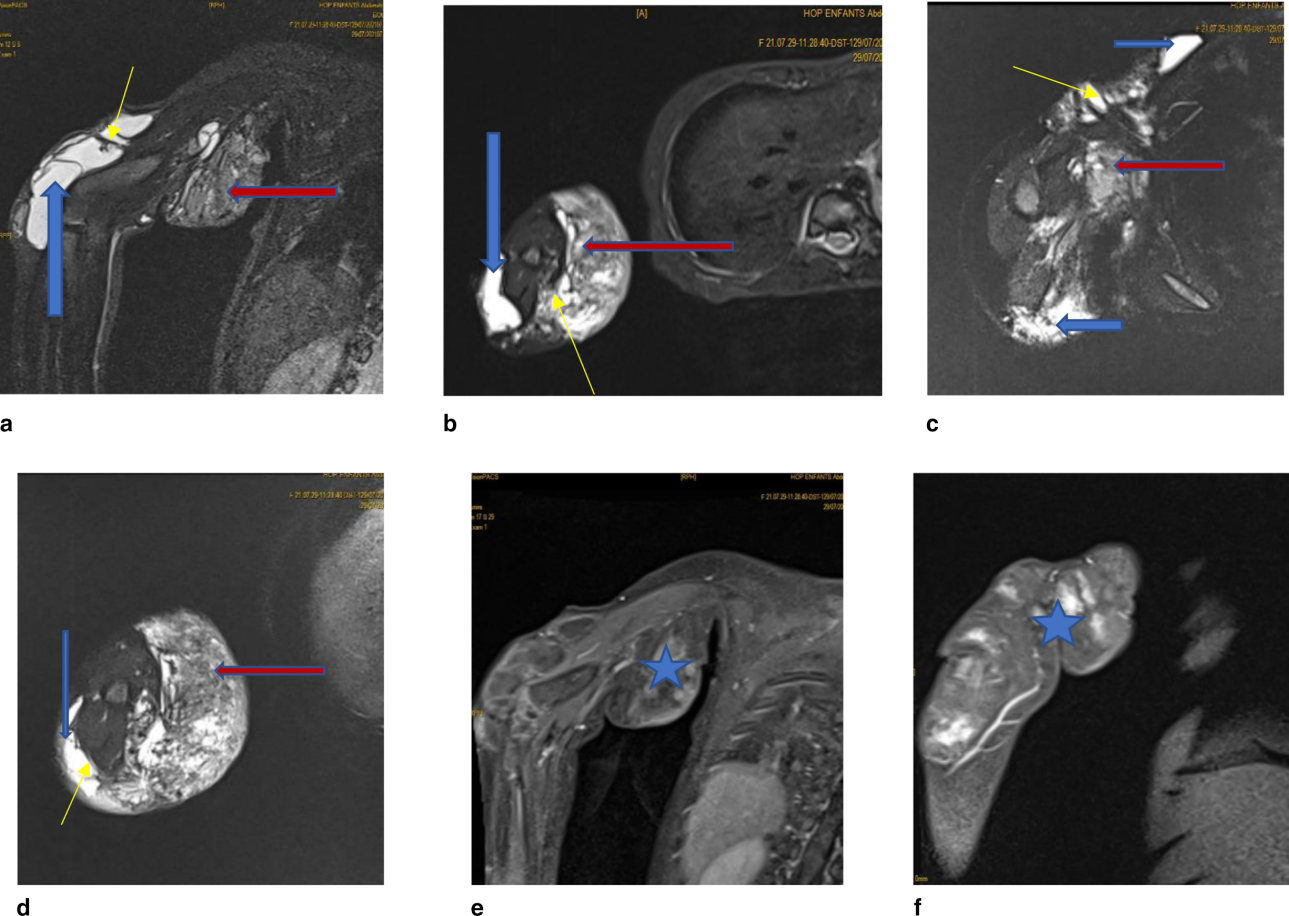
MRI of the right upper limb: cystic lymphangioma of the right axillary soft tissues extended to the arm and the upper third of the forearm with vascular involvement and extension to the thorax. (a) coronal TIR sequence, (b) axial sequence STIR, (c) axial T2 FATSAT sequence, (d) axial T2 FATSAT sequence, (e) and (f) T1 sequence with gadolinium injection. Formation containing multiple cystic logettes of pure liquid signal, of variable size, some of which are macrocystic (red arrows) and others of microcystic appearance (blue arrows). They have hyposignal septa (yellow arrows) on all sequences. They are mostly subcutaneous with intra-muscular extension and encompassing of the vascular pedicle (blue stars) in the axilla and humerus.
